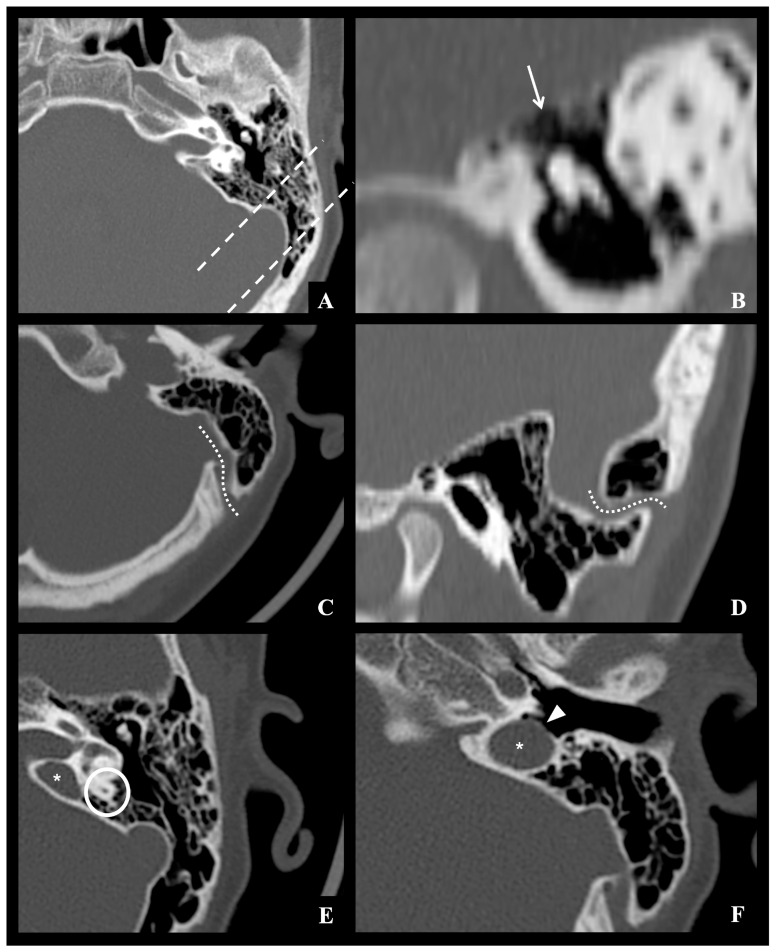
Example of normal mastoid anatomical variants observed on the left side in a same 11-year-old male control patient undergone brain CT examination due to traffic injury: (A) grade IV mastoid hyperpneumatization according to Han et al., with air cells extending beyond the sigmoid sinus posteriorly (dashed lines); (B) thinned tegmen tympani (white arrow); (C,D) accessory mastoid emissary vein enlargement (dotted lines); (E,F) high riding jugular bulb type 4 according to Manjila et al. classification (star), located above the inferior margin of the posterior semicircular canal (white circle), with dehiscent sigmoid plate protruding into middle ear (white arrowhead).
